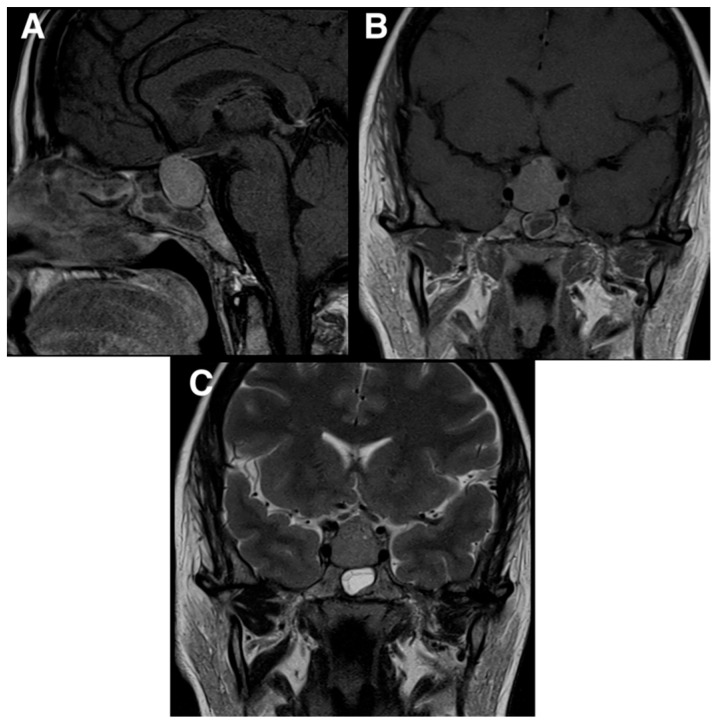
Preoperative MRI scans demonstrating a homogenous mass in the pituitary gland. The MRI also showed that the mass was compressing the optic chiasm and was encasing the carotid arteries. (A) T1 MRI sagittal view; (B) T1 MRI coronal view; (C) T2 MRI coronal view.
